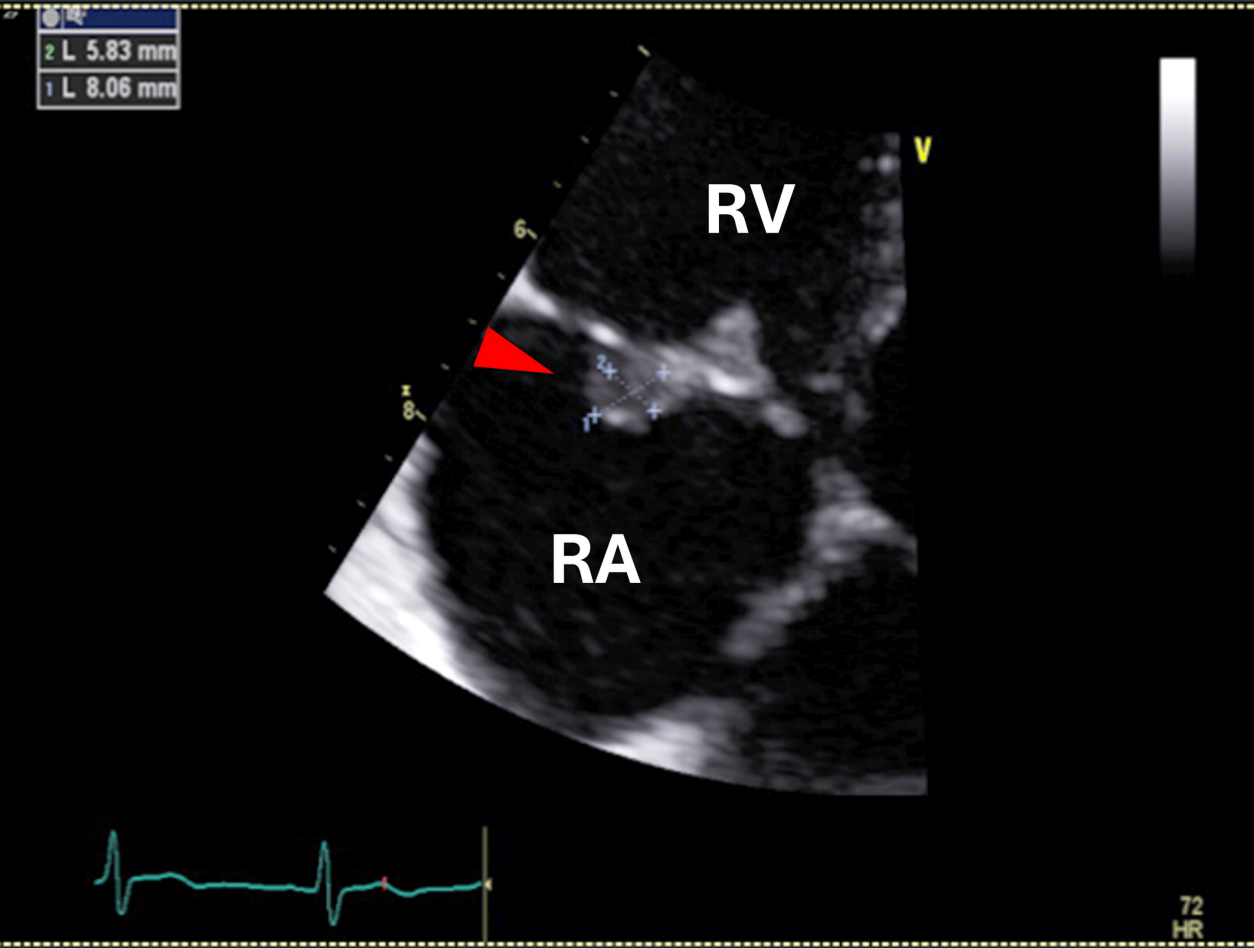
TTE on the day of admission. Repeated TTE on the day of admission showed an 8.0 X 5.8 mm mobile vegetation (arrowhead) on the tricuspid valve. RA: right atrium; RV: right ventricle; TTE: transthoracic echocardiography.
